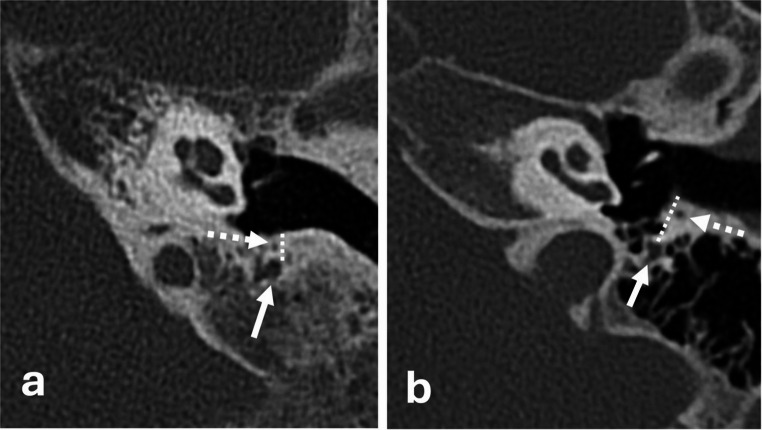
Axial measurement of facial recess width (FRW). Axial CT showing FRW (dashed lines) between the facial nerve canal (solid arrows) and the posterior wall of external auditory canal at the level of round window (RW) and cochlear basal turn. A narrow facial recess (a) (1.5 mm) is associated with increased difficulty of posterior tympanotomy and reduced RW visibility, when compared to a normal FRW (b) (4 mm). Chorda tympani (dashed arrows) are also identified
